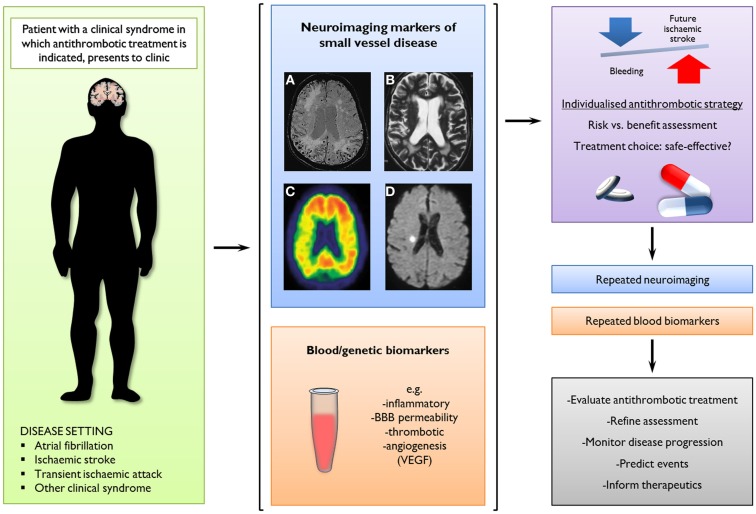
A potential new clinical paradigm of the future role of neuroimaging markers of small vessel disease and blood/genetic biomarkers to predict intracerebral hemorrhage (ICH) by guiding stratified antithrombotic treatment decisions. In this scenario, a combination of imaging markers could be used to assess the relative balance of risk for future ischemic stroke or ICH, informing therapeutic decisions as well as potentially evaluating treatment effects and monitoring disease progression. Neuroimaging modalities and findings with potential clinical implications include: (A) cerebral microbleeds on axial T2*-weighed gradient-recalled echo or susceptibility-weighted imaging; (B) white matter changes on axial T2-weighted MRI; (C) amyloid-b load on PET images using radioligands (e.g., Pittsburgh compound B); and (D) small acute ischemic lesions (possibly cerebral microinfarcts) on axial diffusion-weighted images.
